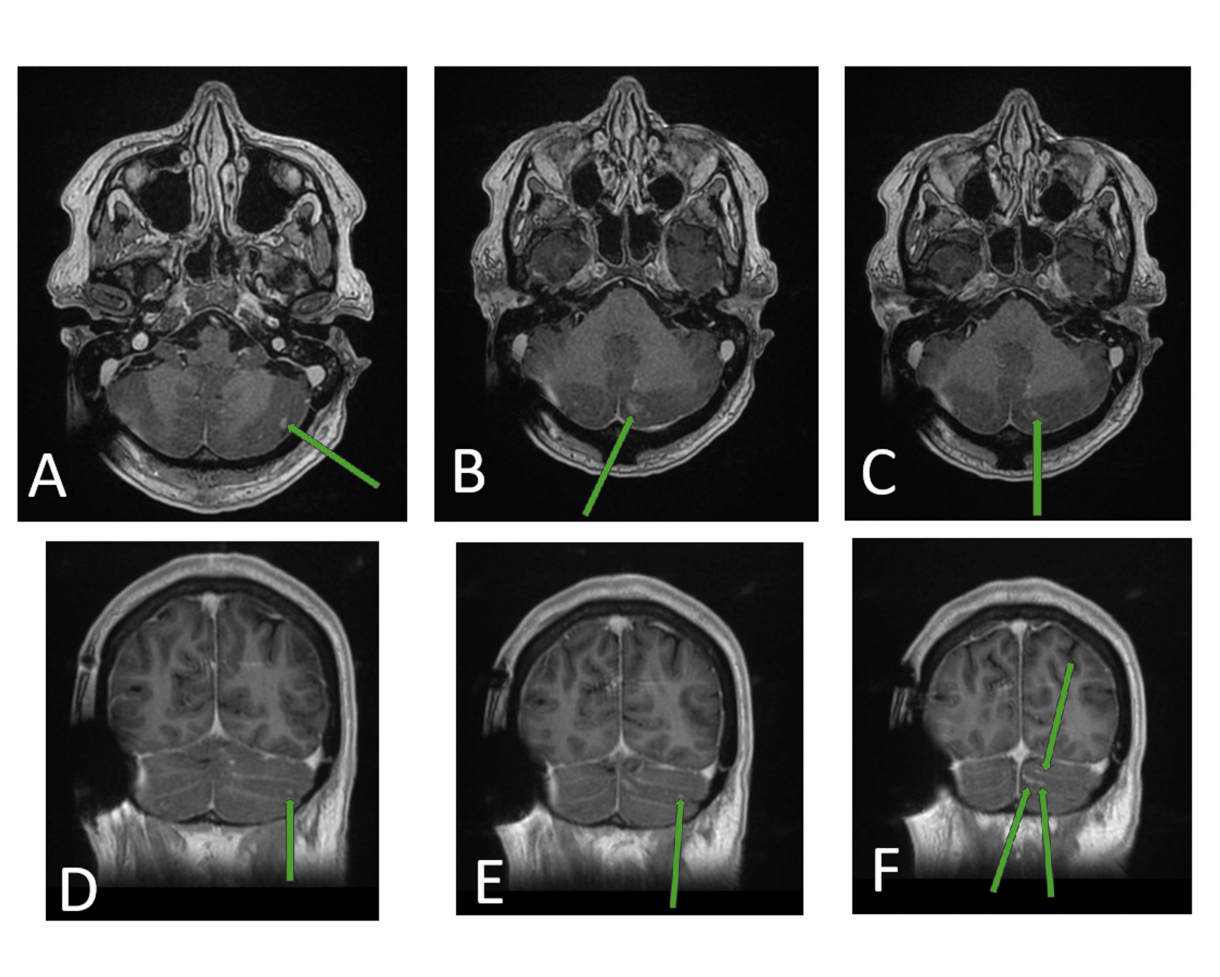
MRI Brain and Spine T1 With Contrast Showing Small Volume Leptomeningeal Enhancement in the Posterior Fossa (A–F) A–C: Axial MRI (T1 with contrast) with progressively caudal slices showing small volume leptomeningeal enhancement in the posterior fossa D–F: Coronal MRI (T1 with contrast) with progressively posterior slices showing small volume leptomeningeal enhancement in the posterior fossa MRI: magnetic resonance imaging
